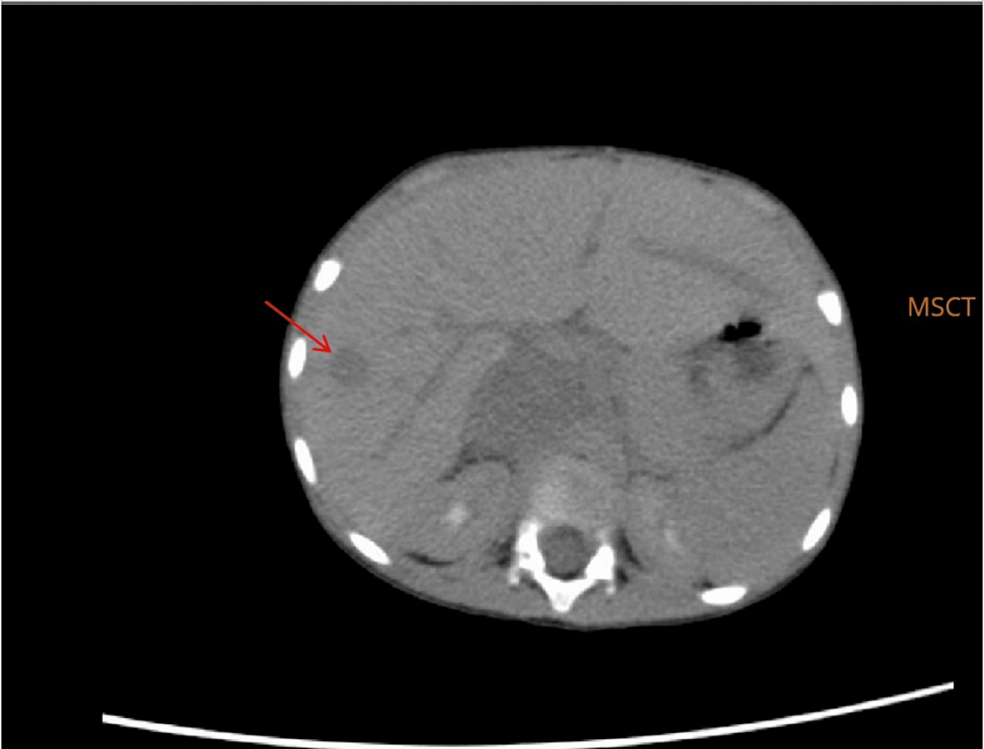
MSCT showed hepatic metastasis. The red arrow showed a hypodense lesion in the segment VI of the liver that is round in shape with irregular borders. MSCT, multislice computed tomography
